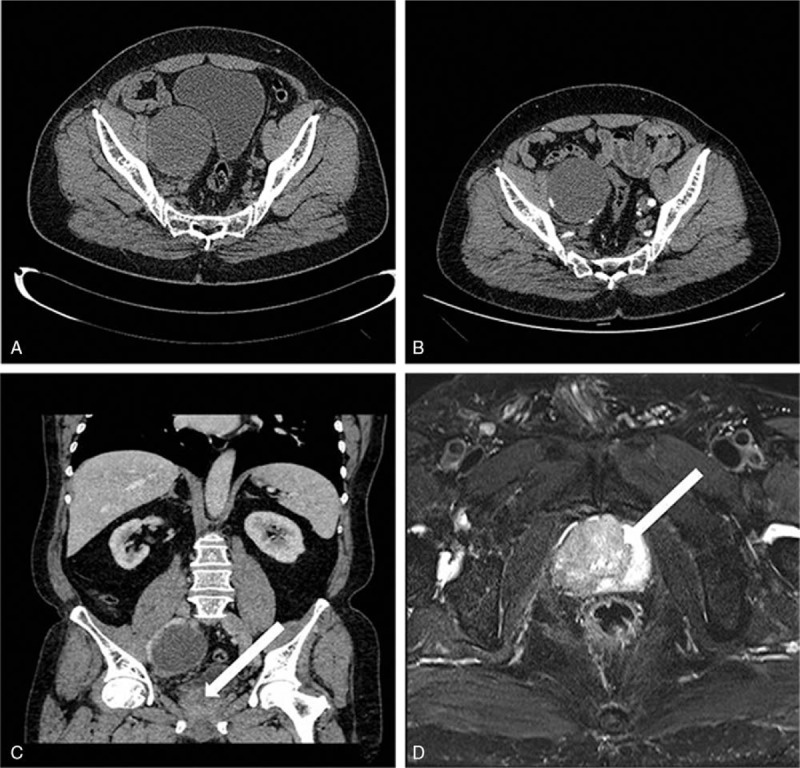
Abdominal CT scanning and MRI of the prostate. (A, B) The nonenhanced and arterial phase of abdominal CT axial scanning shows that a watery low density was located adjacent to the right iliac vessel. There was no obvious enhancement of the lesion in the arterial phase as well. (C) Venous phase of abdominal CT scanning exhibited that there was no significant enhancement in the cystic lesion of right lower abdomen. The enhancement of right part prostate is slightly obvious. (D) Fat-suppressed T2-weighted sequence presents low signal in the right portion of prostate. CT = computed tomography, MRI = magnetic resonance imaging.
